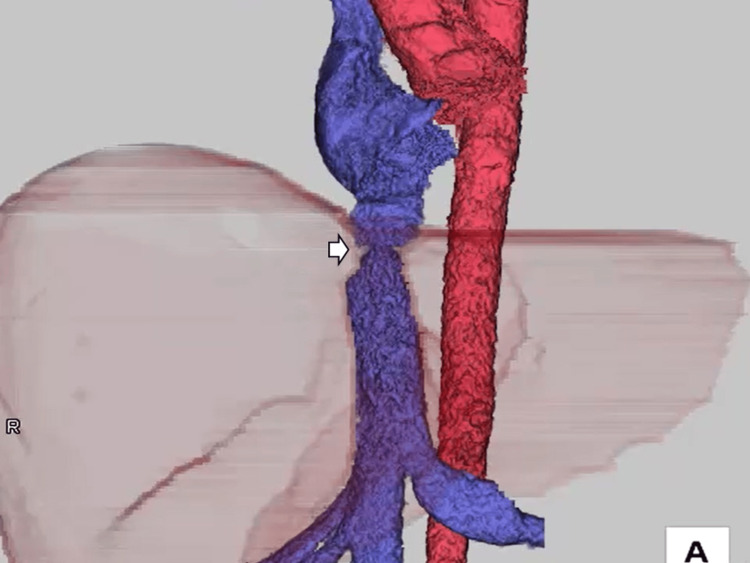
The contrast-enhanced CT scan indicates stenosis of the inferior vena cava (IVC), indicated by the arrow.
